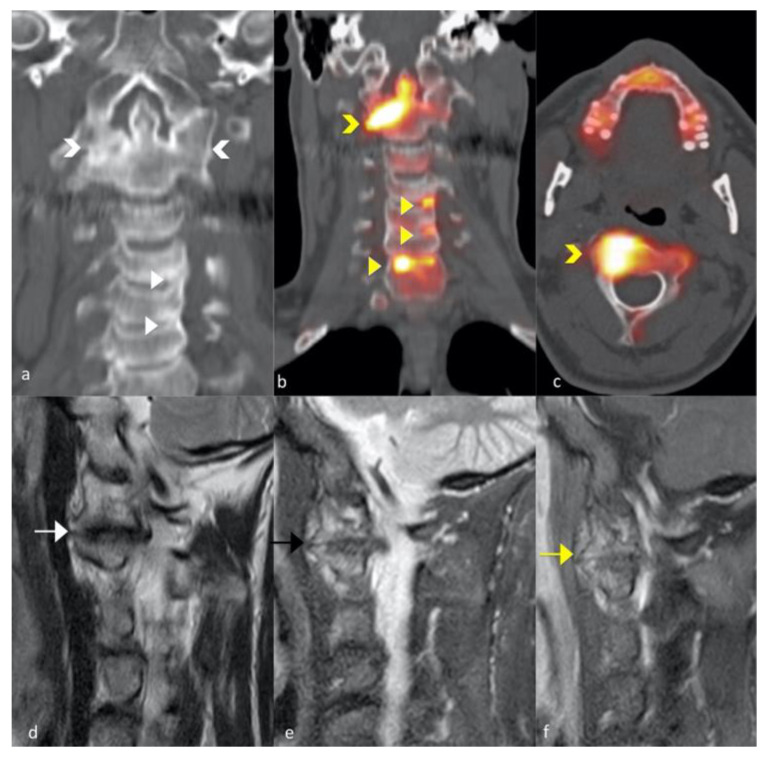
A 56-year-old man with axial PsA. CT coronal image of the cervical spine (a) shows the fusion of both atlanto-axial articulations; huge horizontal oriented osteophytes with extensive sclerosis of the articular cortical bone was observed at the right atlanto-axial articulation. Sodium 18F-Fluoride PET-CT of the cervical spine on the coronal (b), axial (c), and saggital plane (d) shows the increase in tracer uptake at the dens in extension to right lateral mass (yellow chevron); focal areas with increase in tracer uptake were observed at the antero-lateral corners on the left side of C3-C4 and C4-C5 levels and on the right antero-lateral corner of the vertebral plate of C7 (yellow-arrow-heads); the corresponding CT image (a; arrowheads) shows signs of structural damages with bone sclerosis also with small cortical erosions. Sagittal T2-weighted and short tau inversion recovery (STIR) T2-weighted TSE images (d,e) show signs of structural damages at the right atlanto-axial articulation with bone formation and bone oedema with the involvement of periarticular soft tissues (arrows); sagittal fat-suppressed gadolinium-enhanced T1-weighted FS TSE (f), show contrast enhancement of articular space, also involving cortical bone and periarticular soft tissue, in keeping with active synovitis (yellow arrow).
